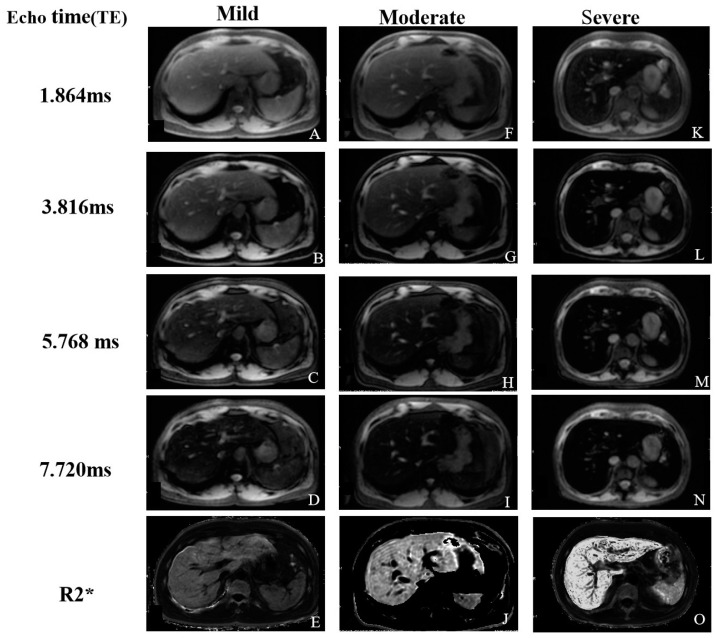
In three patients with different degrees of liver iron overload (mild, moderate, and severe), the provided images consisted of axial gradient-echo (GRE) MR images acquired at 3.0 T, utilizing multiple echo times (TEs) (1.864, 3.816, 5.768, and 7.720 ms). These images are accompanied by corresponding R2* maps. (A–E): Mild iron overload in a 45-year-old man, using precise quantitative MRI techniques, T2* = 3.018 ms, R2* = 274.1 Hz. (F–J): Moderate iron overload in a 47-year-old man, T2* = 3.012 ms, R2* = 283.9 Hz. (K–O): Severe iron overload in a 53-year-old woman, T2* = 1.059 ms, R2* = 721.6 Hz. Iron overload in the liver parenchyma leads to a decrease in T2* and an increase in R2* values. As the degree of liver iron overload increases, there is a corresponding intensification of T2* reduction and R2* elevation in three cases.
